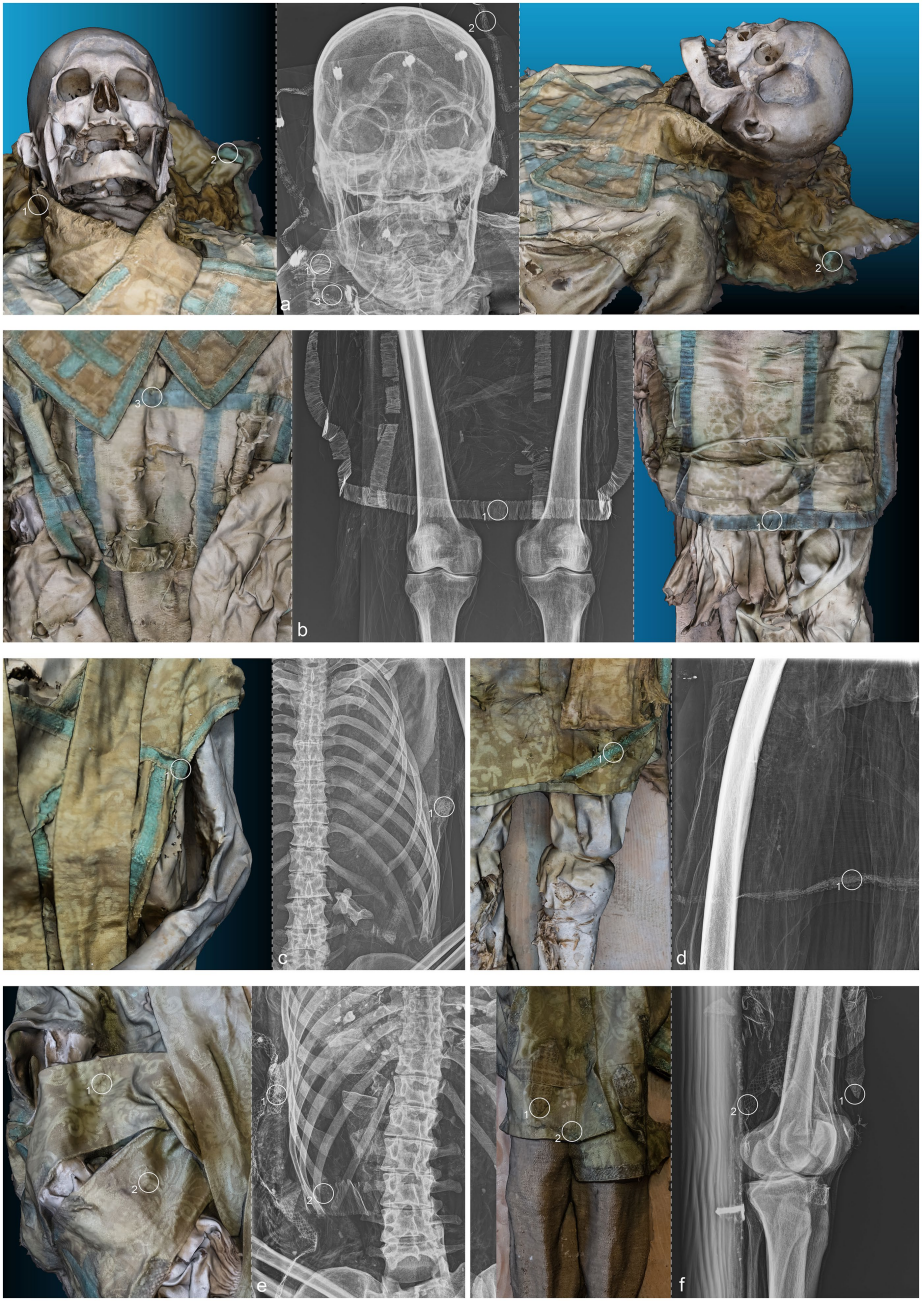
(a) Anteroposterior radiograph of the skull of ID1; (b) Anteroposterior radiograph of the lower limbs of ID1; (c) Anteroposterior radiograph of the thorax of ID2; (d) Anteroposterior radiograph of the right femur of ID2; (e) Anteroposterior radiograph of the thorax of ID3; (f) Lateral radiograph of the lower limbs of ID3. The three-dimensional reconstructions, separated by a dashed line from the radiographic acquisitions, correspond to the radiographed anatomical areas and are numbered accordingly.
